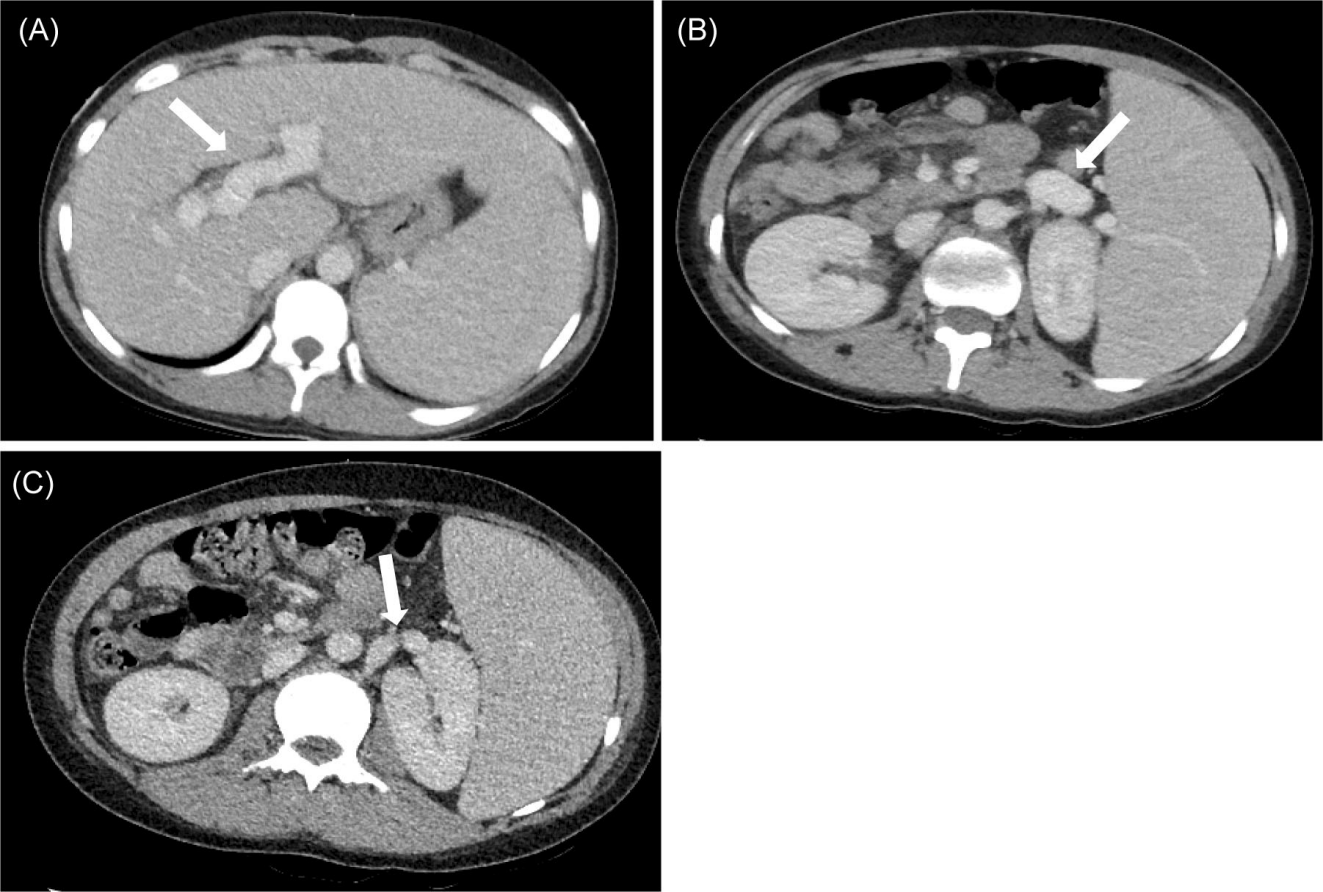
Abdominal CT scan findings of portal hypertension. (A) Axial CT with IV contrast demonstrates enlarged intrahepatic portal vein (arrow) with splenomegaly; (B) large splenic hilum varix (arrow); (C) varix drains to left renal vein as a splenorenal shunt (arrow). CT, computed tomography; IV, intravenous.
